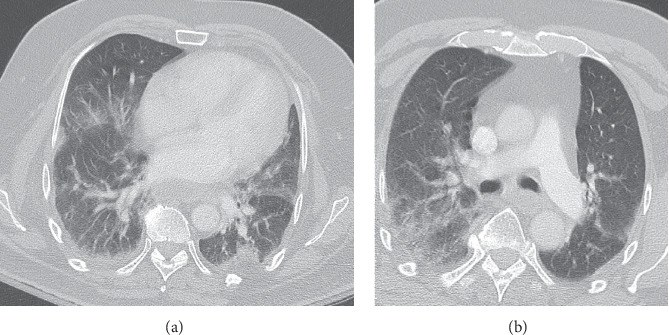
(a) Subpleural and peripheral interstitial infiltrate [3–5] which is worrisome for coronavirus or other viral or atypical infections. (b) Computed tomography angiography showed negative for pulmonary embolus but showed signs of subpleural and peripheral interstitial infiltrate suggestive of COVID-19 vs. other viral infections [3–5]. However, prior chest X-ray on admission was negative for any acute changes when compared to previous studies.
